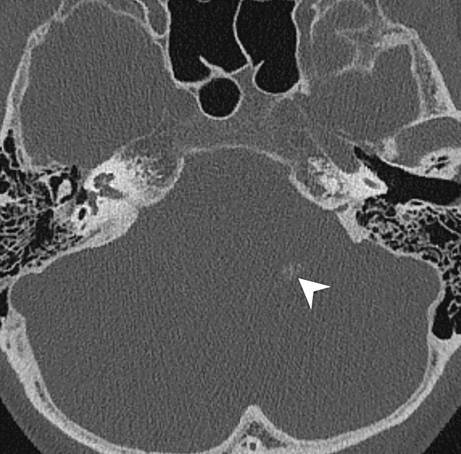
Axial CT image of the posterior fossa in bone window setting displays a sprinkled, sand-like calcification within the lesion (*arrowhead*)
